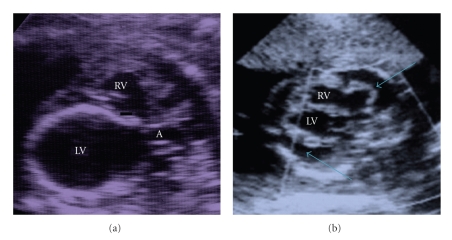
(a) Echocardiography of a fetus with endocardial fibroelastosis—a high echodensity of the left ventricular (LV) walls due to calcifications is evident. RV—right ventricle, A—aorta. (b) Fetus with myocarditis and fetal hydrops: both ventricles and atria are dilated and the arrows indicate the pericardial effusion. RV—right ventricle, LV—left ventricle.
